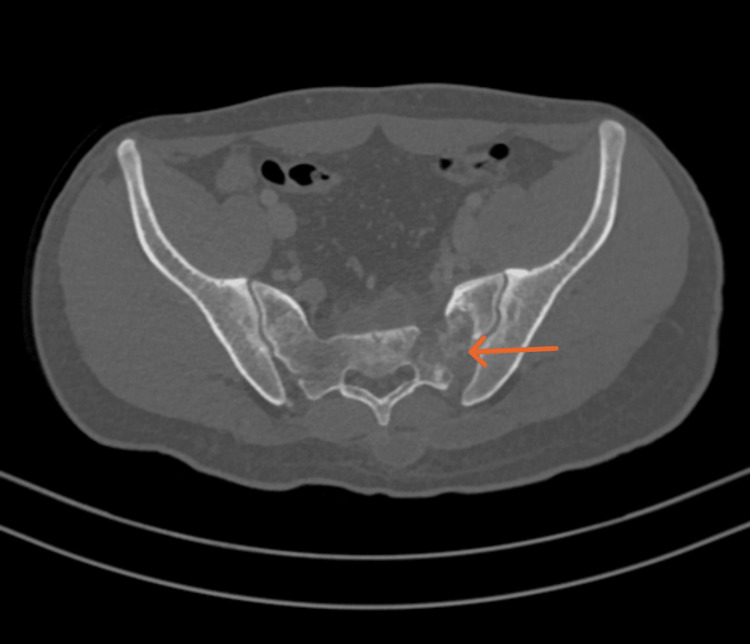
Axial cut CT of the pelvis demonstrating destructive lytic lesion in the left sacral bone.
